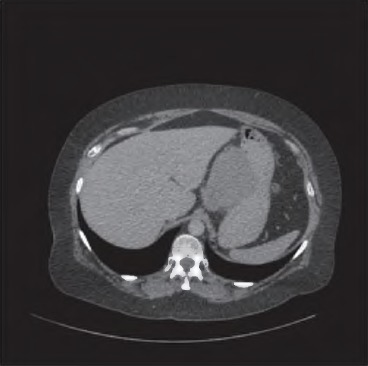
Computed tomography showing the 9cm irregular homogenous lesion arising from the lesser curve of the stomach
